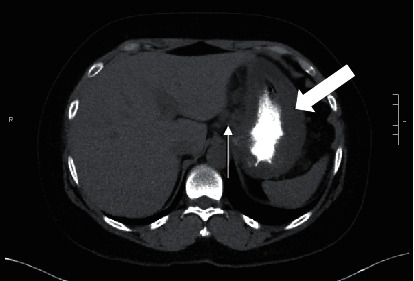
CT scan of the abdomen displaying the transmural thickening of the stomach (white blocked arrow) and the perigastric inflammation with reactive adenopathy (white arrow).
